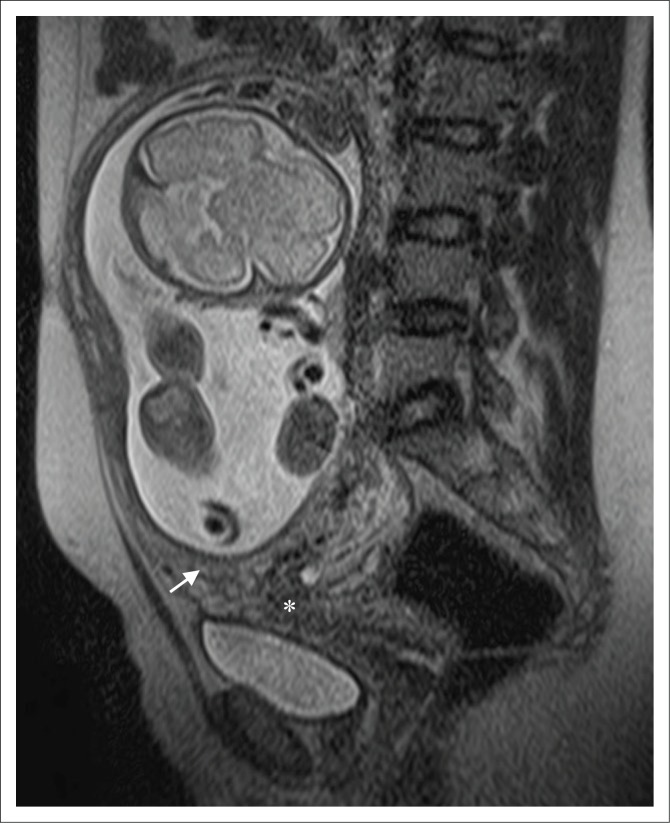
Sagittal T2-weighted magnetic resonance imaging demonstrating low-lying placental tissue (white arrow), separate from the main bulk of the placenta, with complete coverage of the internal ostium (os) of the cervix (asterisks).
